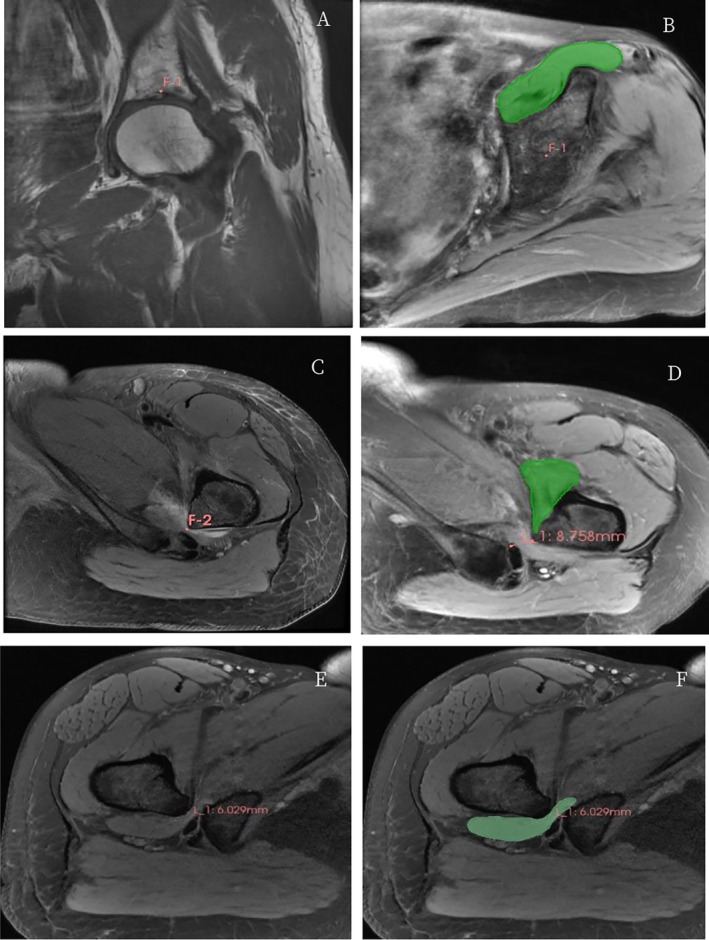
MRI for anatomical delineation of the iliopsoas and quadratus femoris muscles. (A) Proximal border of iliopsoas. (B) Proximal CSA. (C) Distal border. (D) Distal CSA. (E) QFS measurement. (F) QF muscle CSA. Abbreviations: CSA, cross‐sectional area; IFS, ischiofemoral space; QFS, quadratus femoris space; QF, quadratus femoris.
